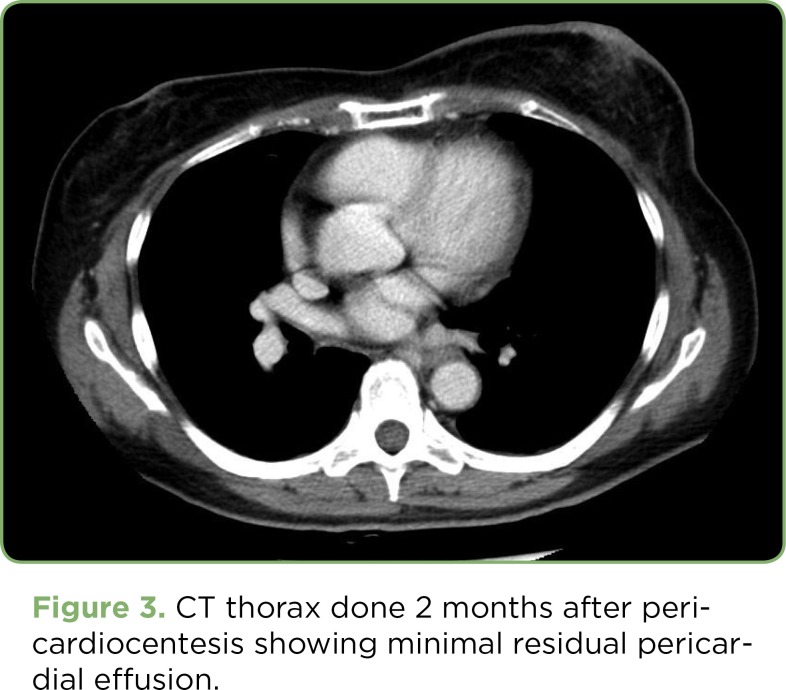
CT thorax done 2 months after peri - cardiocentesis showing minimal residual pericar - dial effusion.
